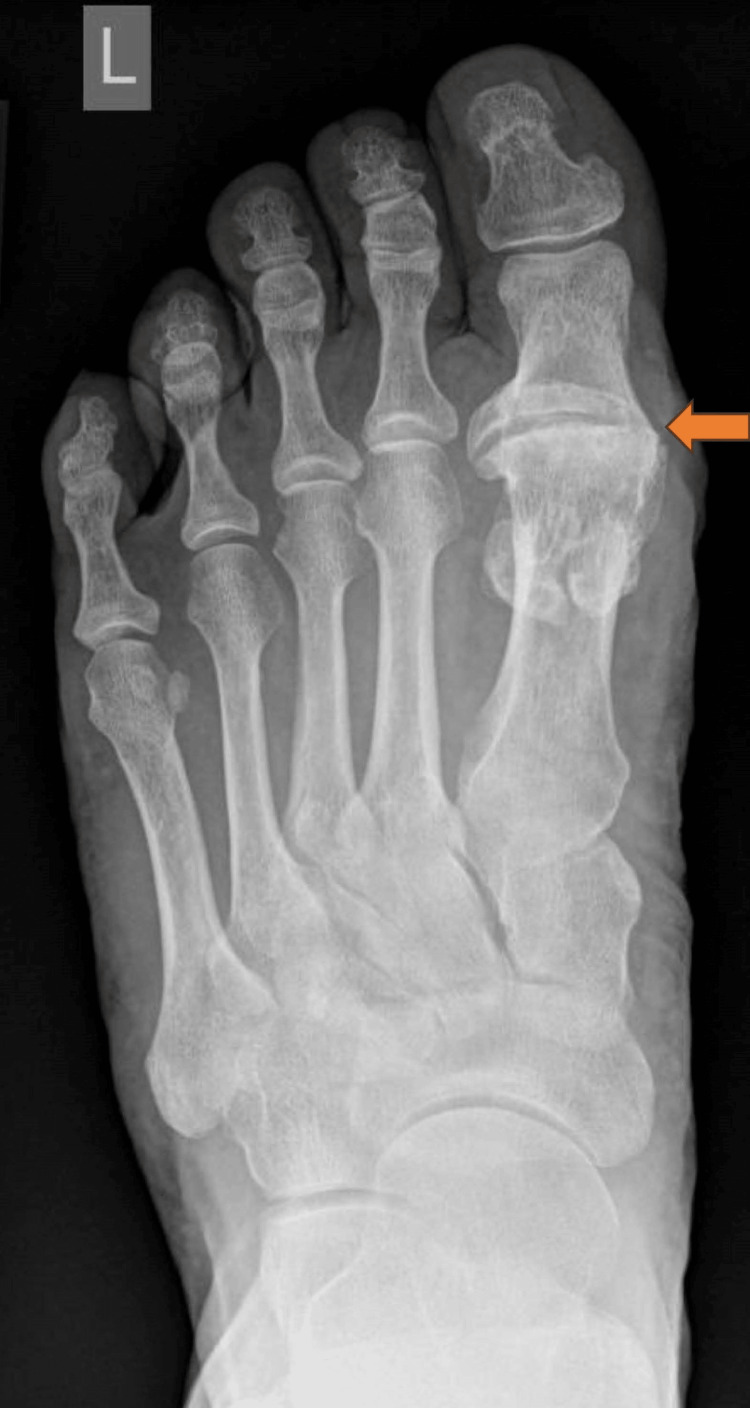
Pre-operative Radiograph Showing First Metatarsophalangeal Joint Arthritis
